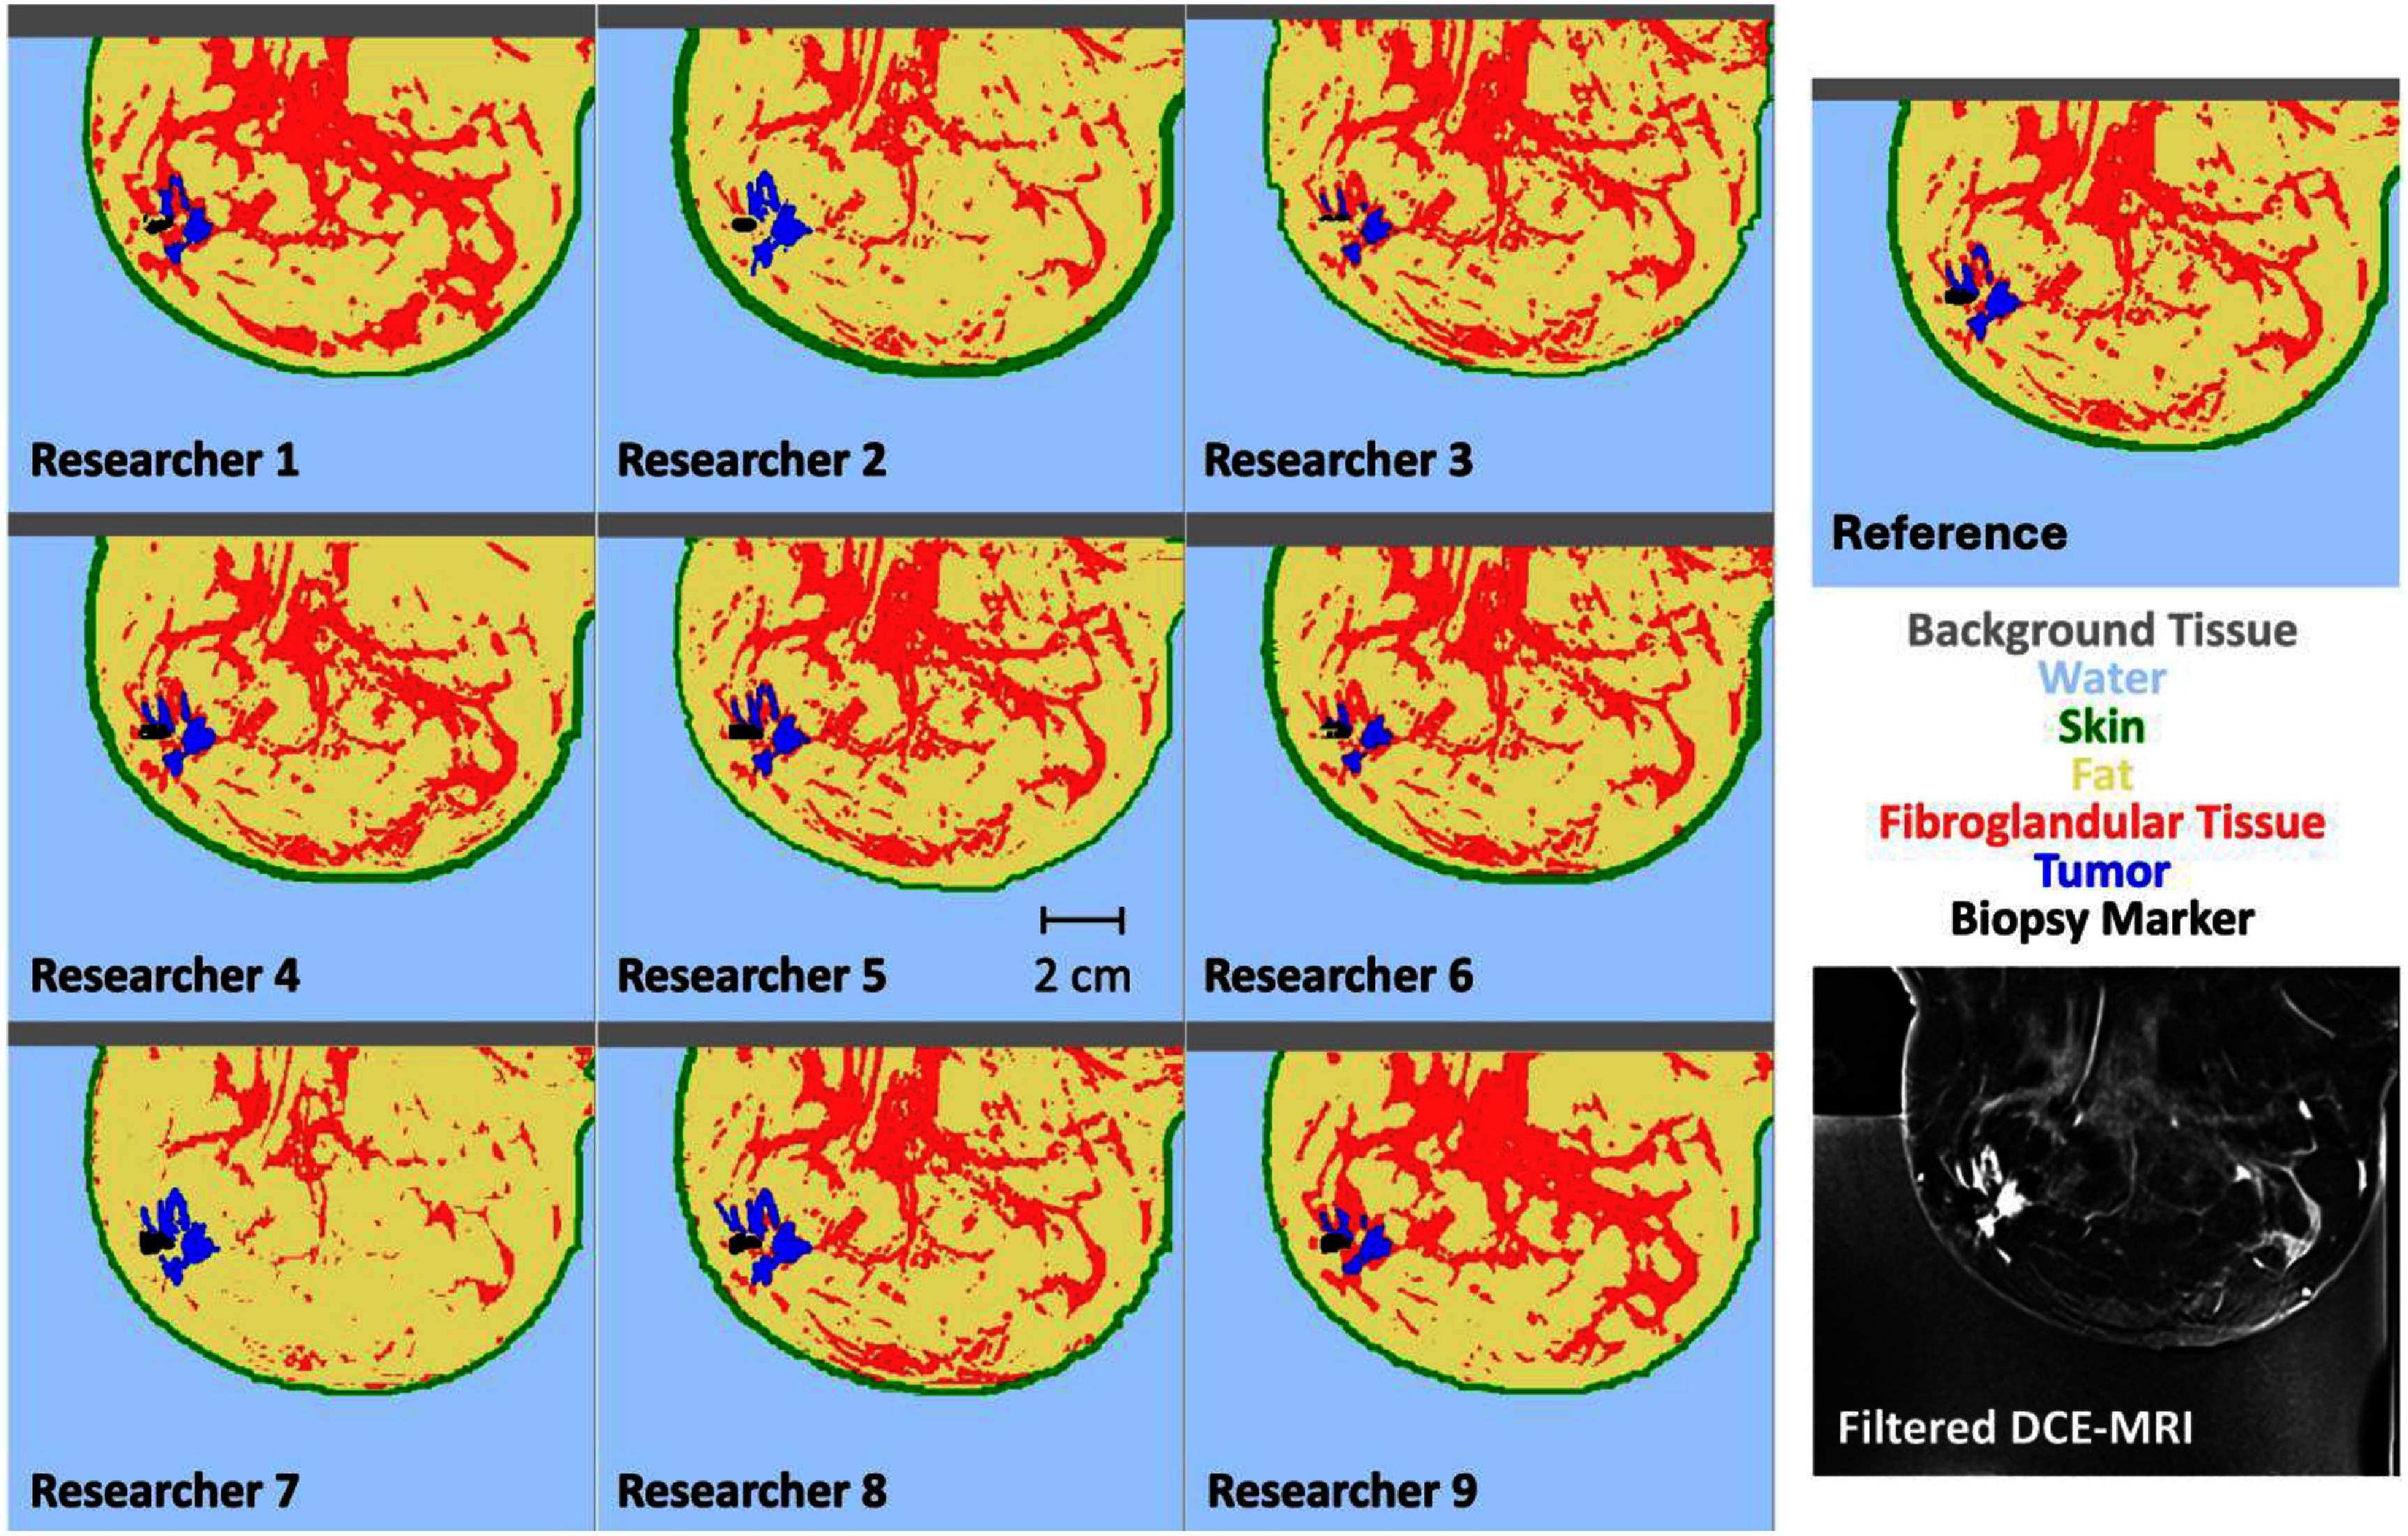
A single cross-section of the Patient 1 segmentation datasets and the corresponding filtered DCE-MRI. Datasets on the left were created by different researchers, and the top right dataset is the reference STAPLE-generated segmentation. Color coding of tissue labels is listed at right.
